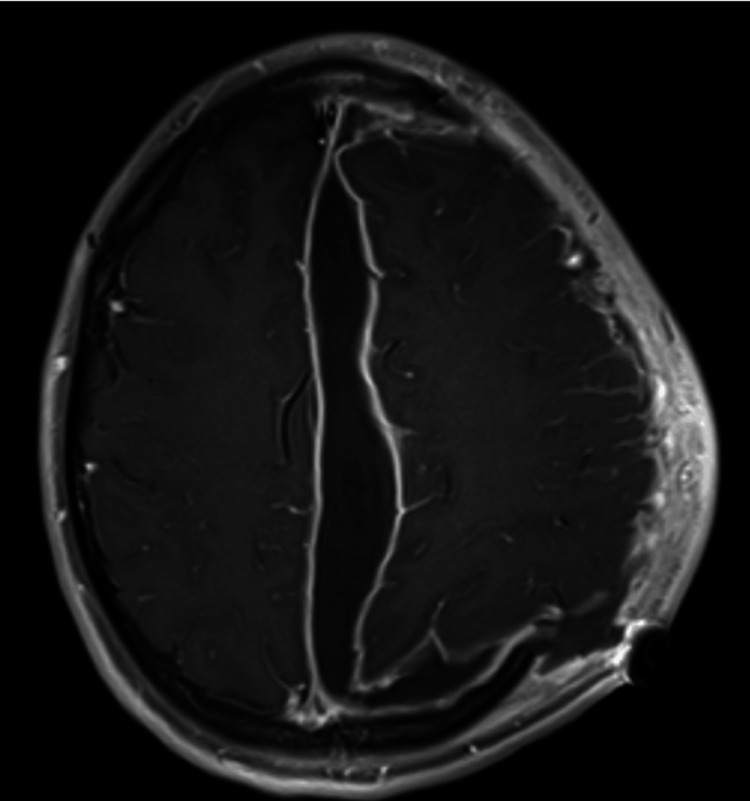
Contrast-enhanced cranial MRI three weeks post-craniectomy. T1 fat-saturated image after intravenous contrast showing a large midline extra-axial fluid collection consistent with relapsed subdural empyema. MRI: magnetic resonance imaging
